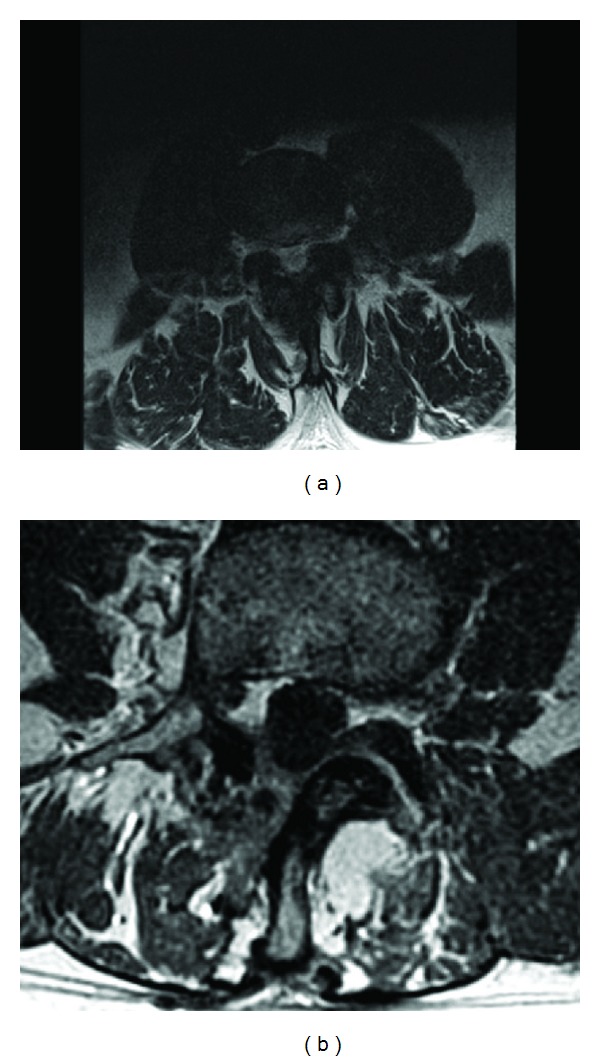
Preoperative (a) and postoperative (b) cross-sectional MRI of lumbar spine demonstrating significant enlargement of thecal sac.
